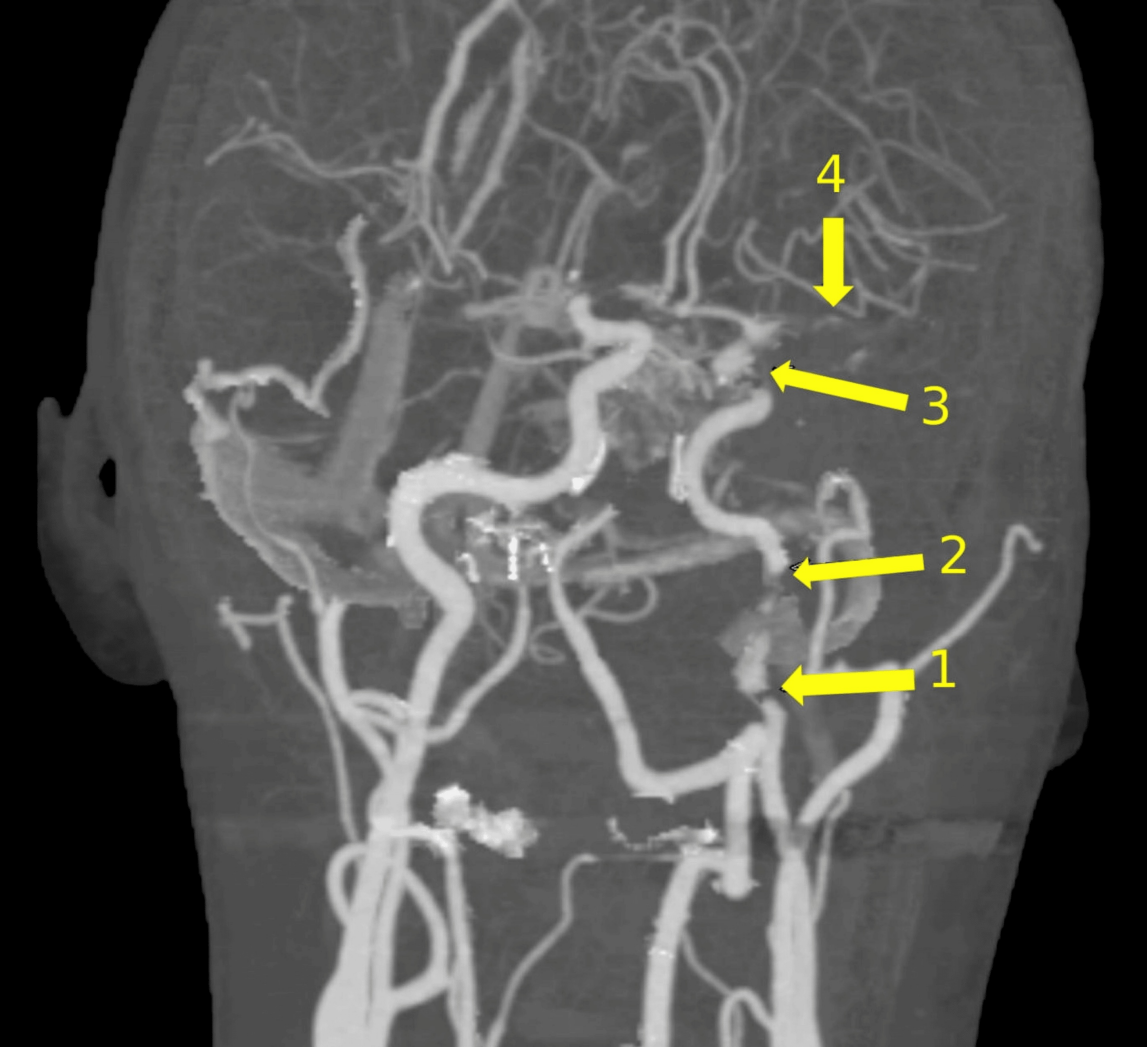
Reconstructed CT angiogram image showing (1) dissection in the distal cervical ICA, (2) refilling of the carotid canal and cavernous part of the left ICA, (3) dissection in the clinoid and supraclinoid ICA, and (4) extension of the dissection in the left MCA
